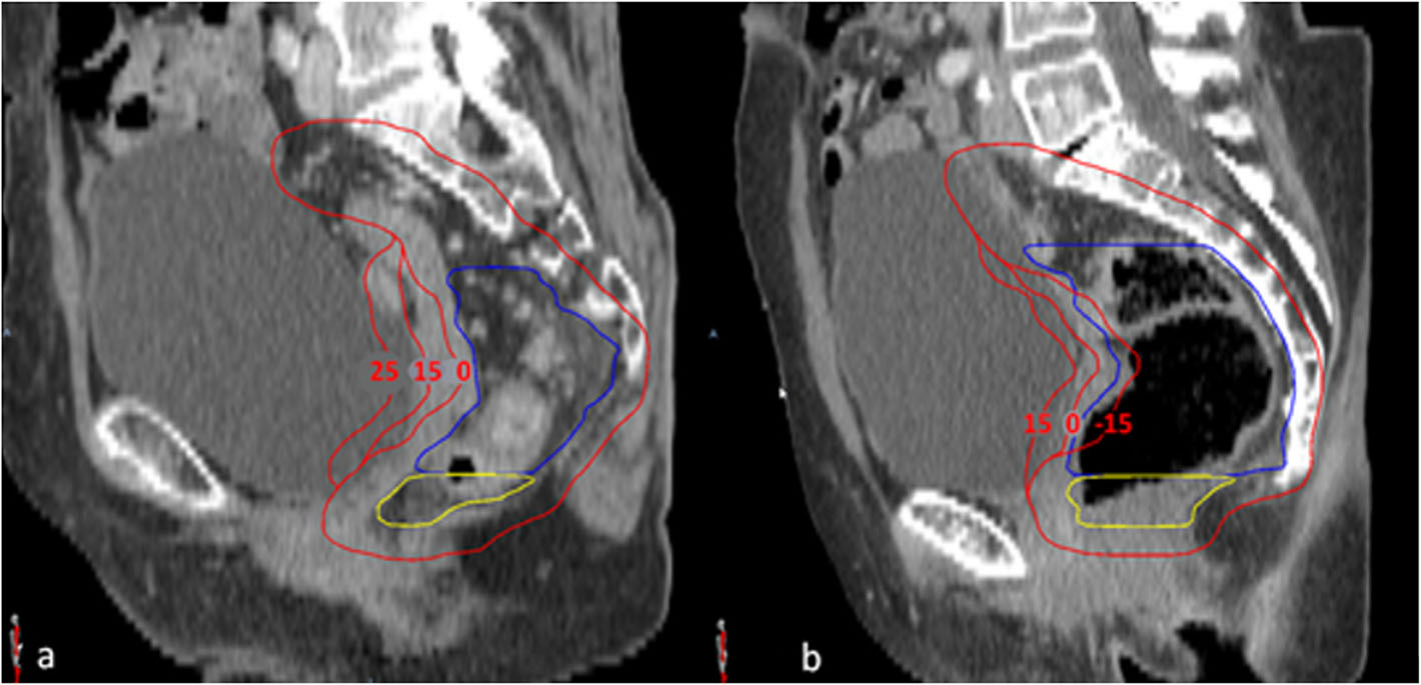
Margin sets based on anatomy as captured on planning CT. a shows an empty rectum with a set of 25 mm, 15 mm, and 0 mm margins (red) for the upper mesorectum (blue). b shows a full rectum with a set of 15 mm, 0 mm, and − 15 mm anterior margins (red) for the upper mesorectum (blue). Yellow is the lower mesorectum
